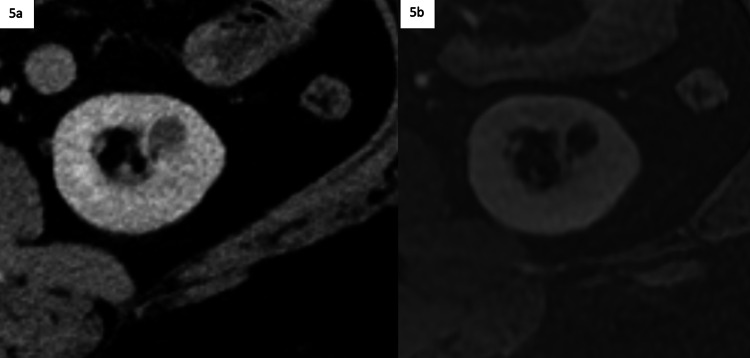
Enhanced abdominal CT with follow-up contrast-enhanced abdominal MRI following admission 5a: Contrast-enhanced abdominal CT ordered in the emergency room for abdominal pain, demonstrating an incidentally detected indeterminate left renal lesion measuring less than 2 cm. 5b: Contrast-enhanced abdominal MRI subtraction image, demonstrating a nonenhancing Bosniak 2 cyst. The MRI was ordered 2 days after the patient was admitted from the ER, during their inpatient stay.
